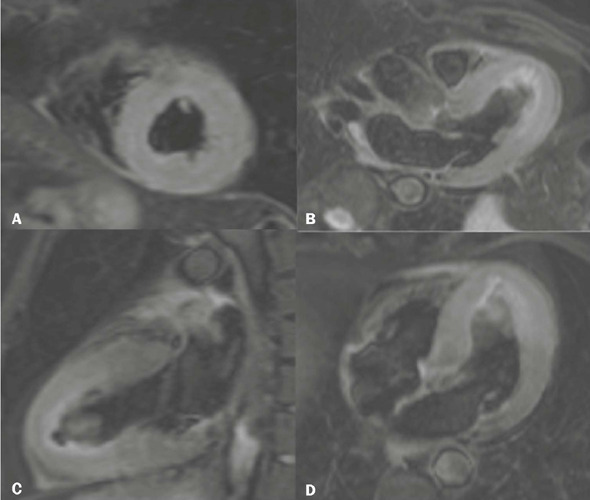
T2-weighted CMR (triple inversion recovery) sequence with fat saturation
